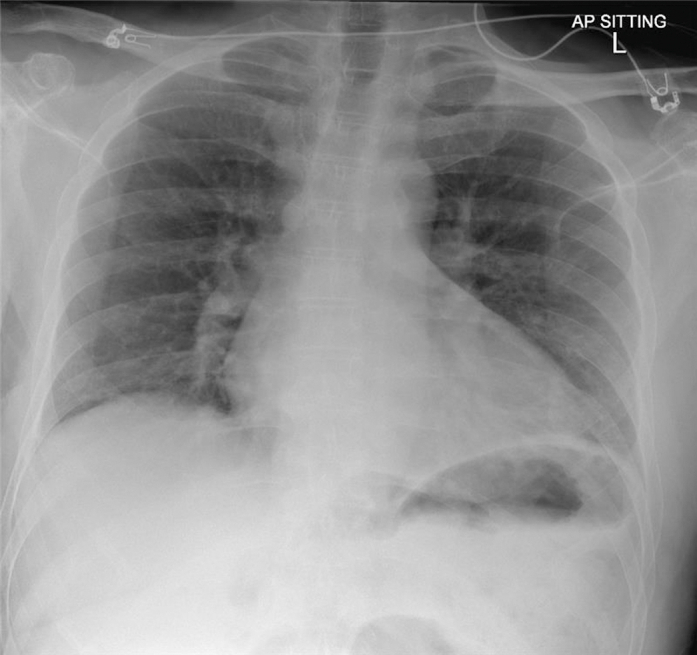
Chest X-Ray Shows underinflated lungs with no evidence of confluent consolidation, pulmonary edema, or pleural effusion.
